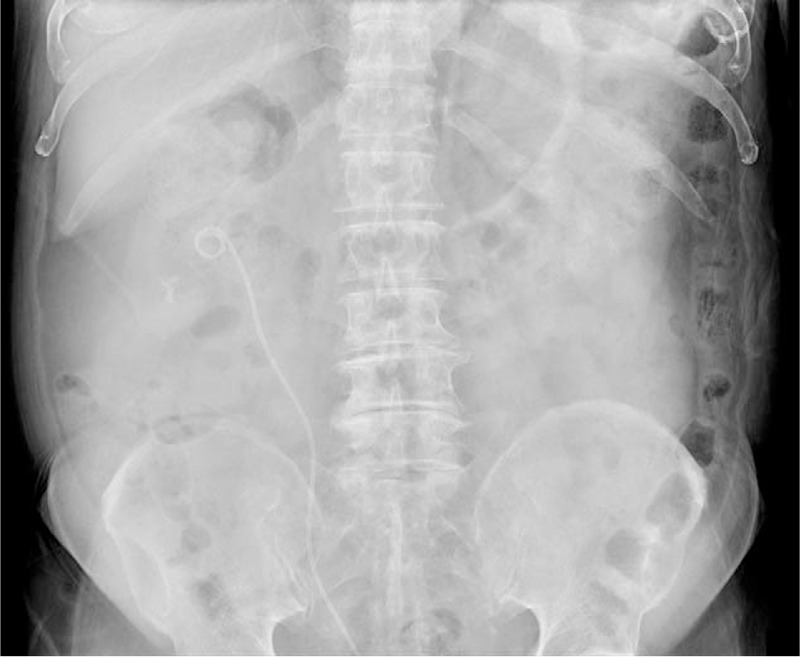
Postoperative kidney, ureter, and bladder radiograph showed no residual stones.
